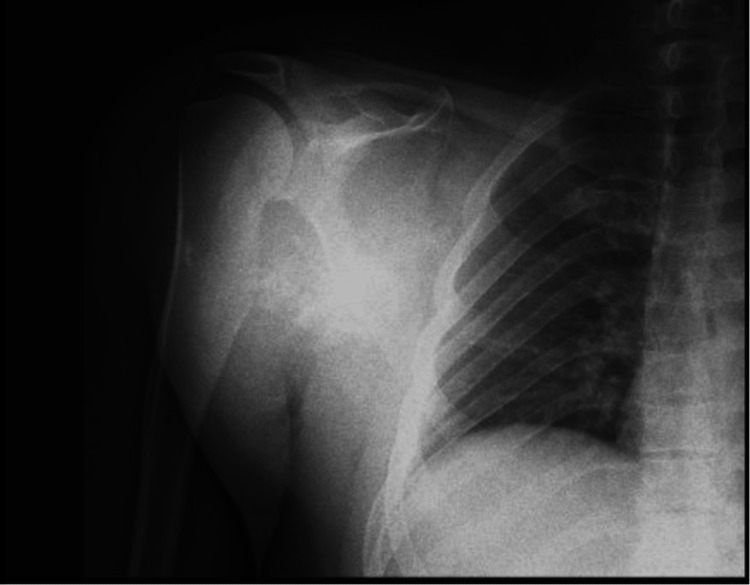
Anteroposterior preoperative X-ray view showing a large soft tissue mass along the chest wall and right axillary region.
